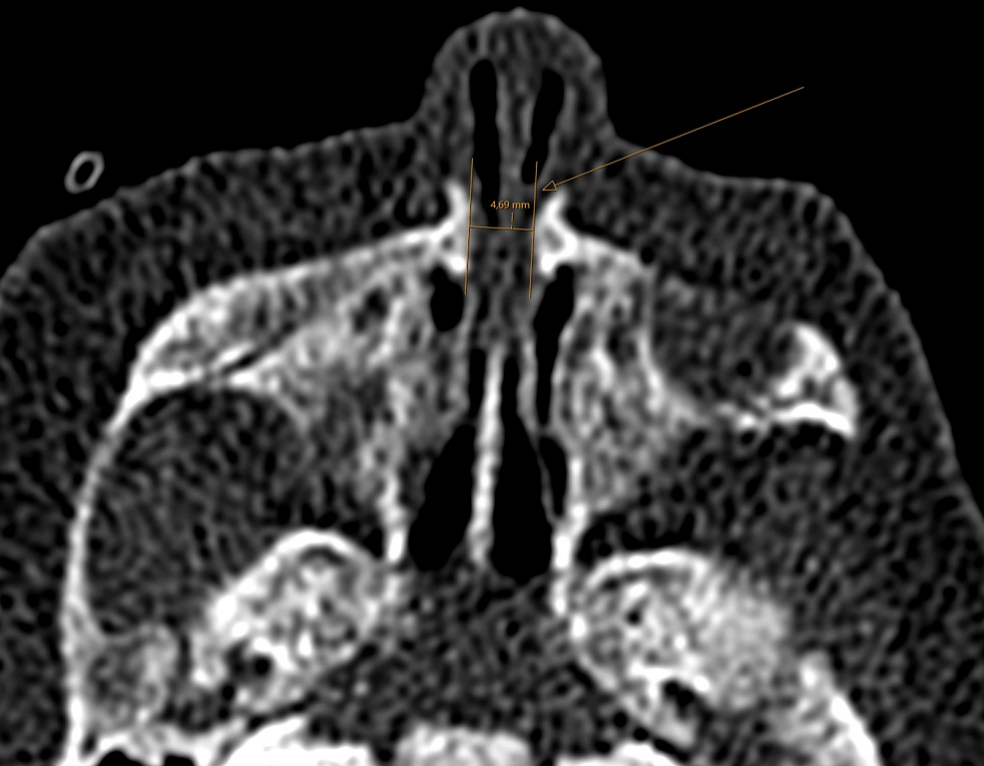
CT scan shows the narrowing of the piriform sinuses in a three-month-old female. The stenosis was 4.7 mm (arrow). CT: computed tomography
